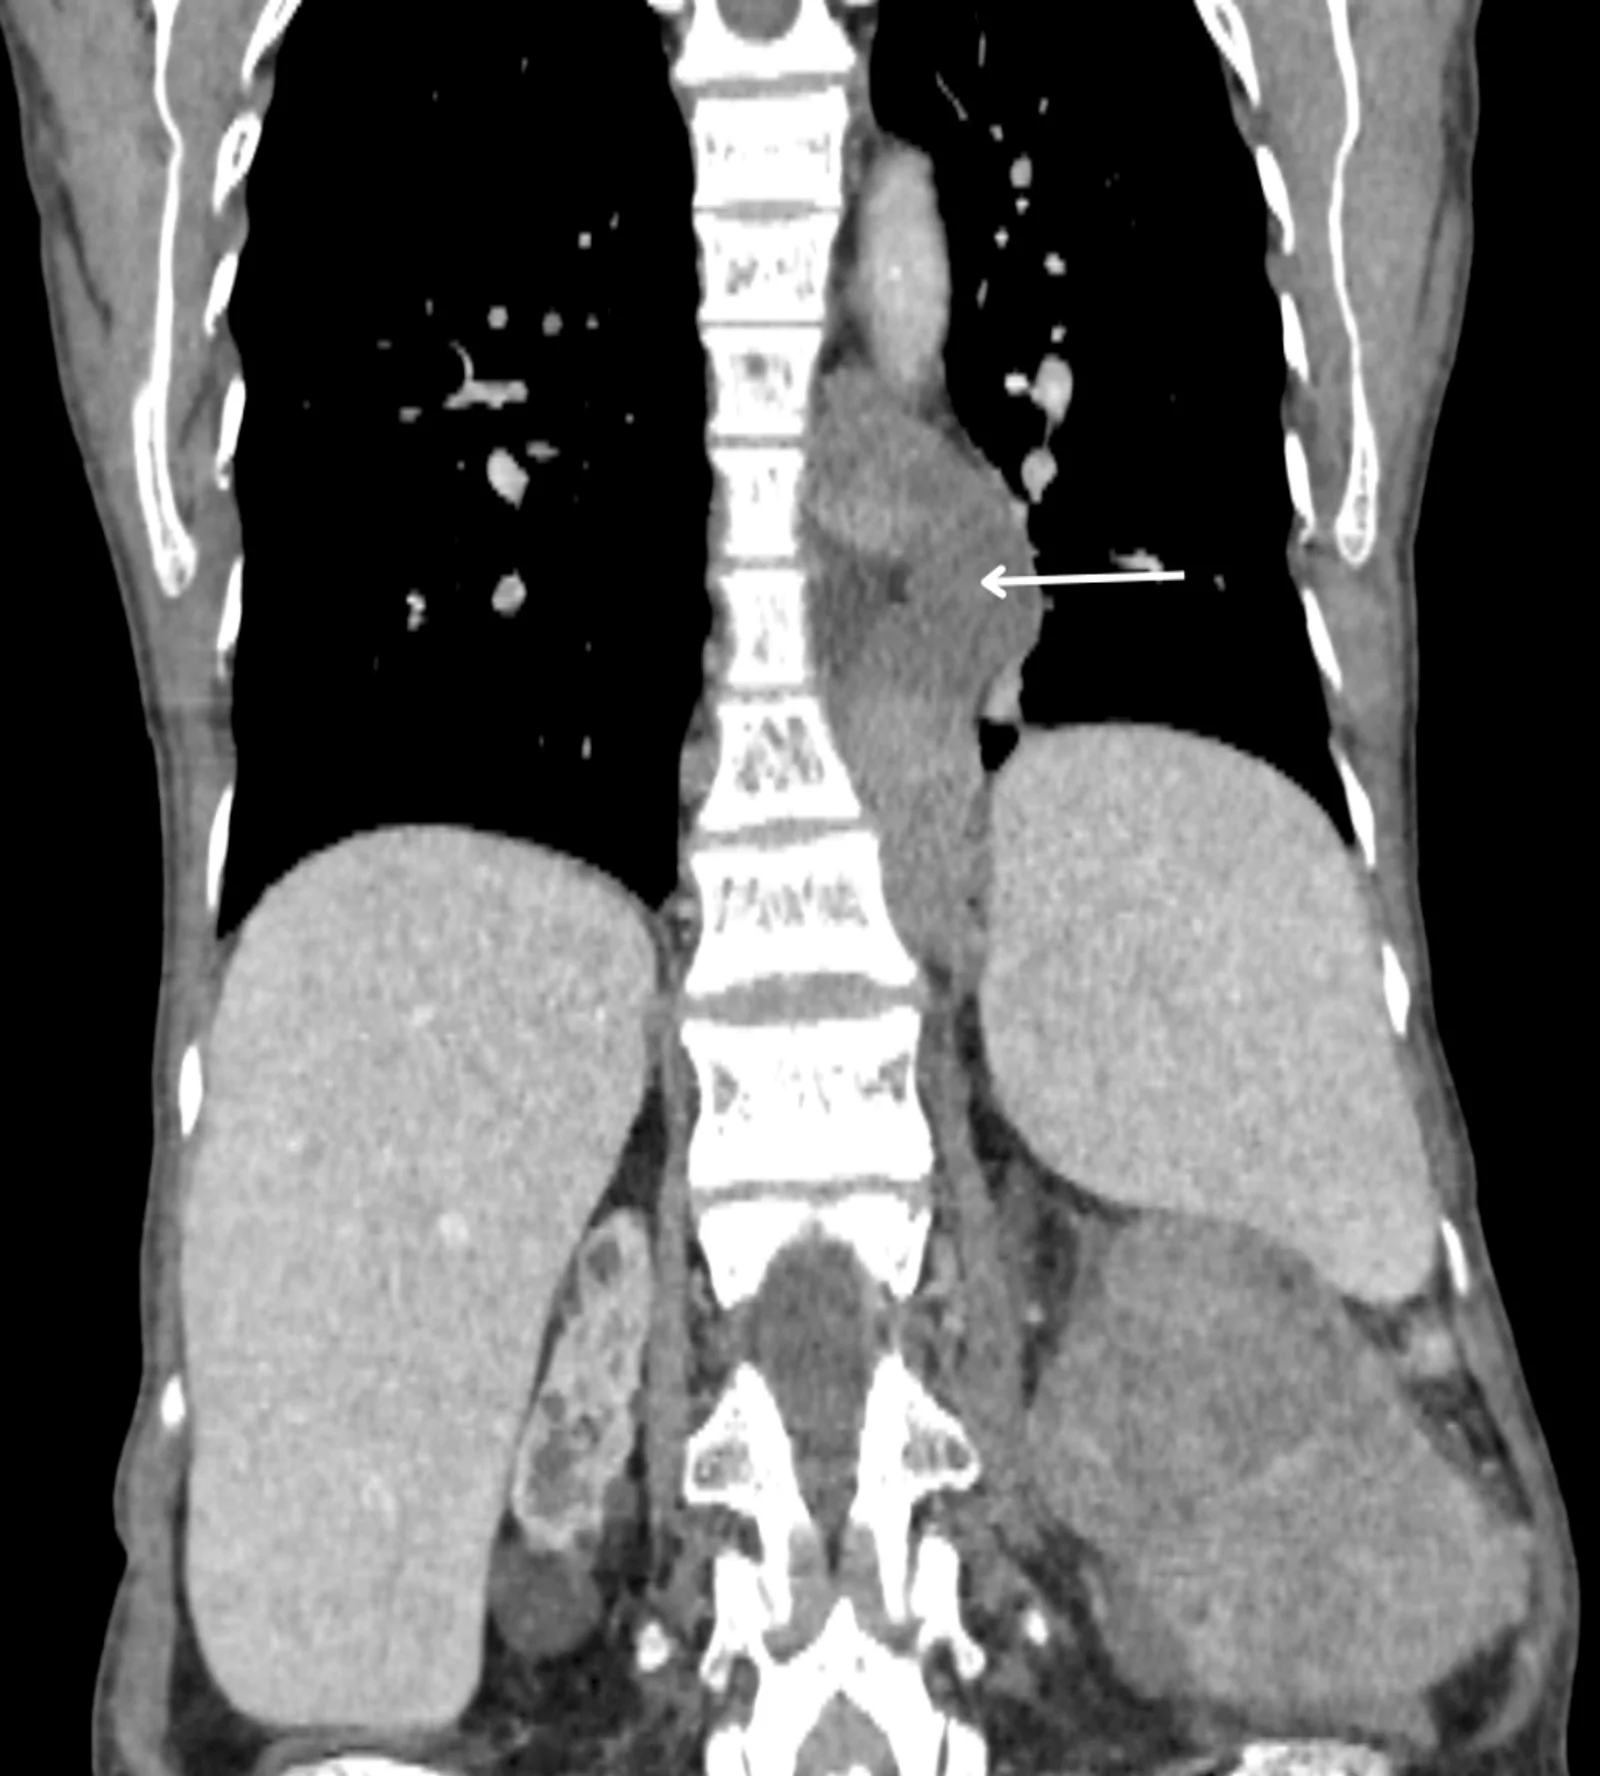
Coronal contrast-enhanced CT reconstruction obtained at a higher thoracic level demonstrating continued tumor thrombus within the left hemiazygos vein (white arrow).
